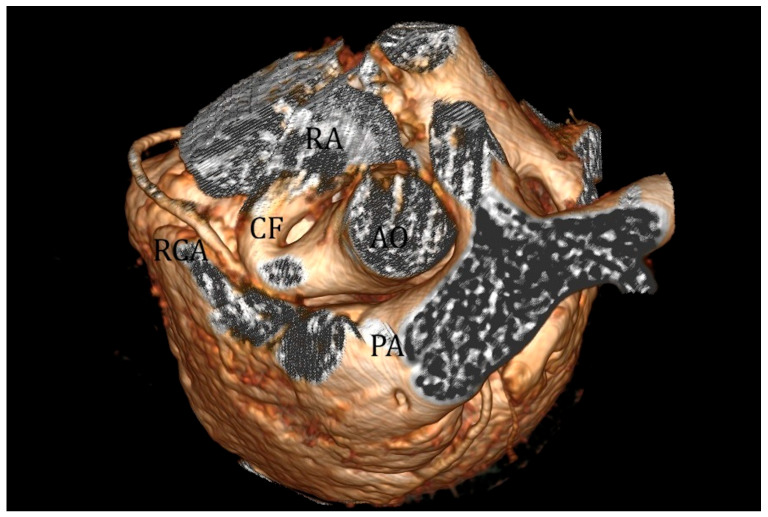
Angio-CT scan three-dimensional view of a large coronary fistula (CF) between the right coronary artery (RCA) and the right atrium (RA). Abbreviations: AO: aorta; PA: pulmonary artery.
